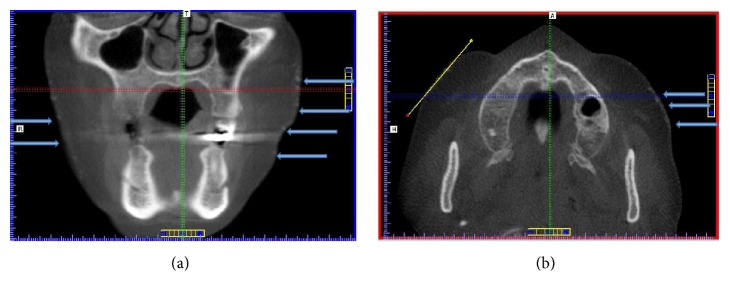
(a) CBCT coronal view. (b) CBCT axial view showing numerous radiopaque small concentric nodules in the soft tissues below the level of the zygomatic arch and lateral to the buccinator muscle.
